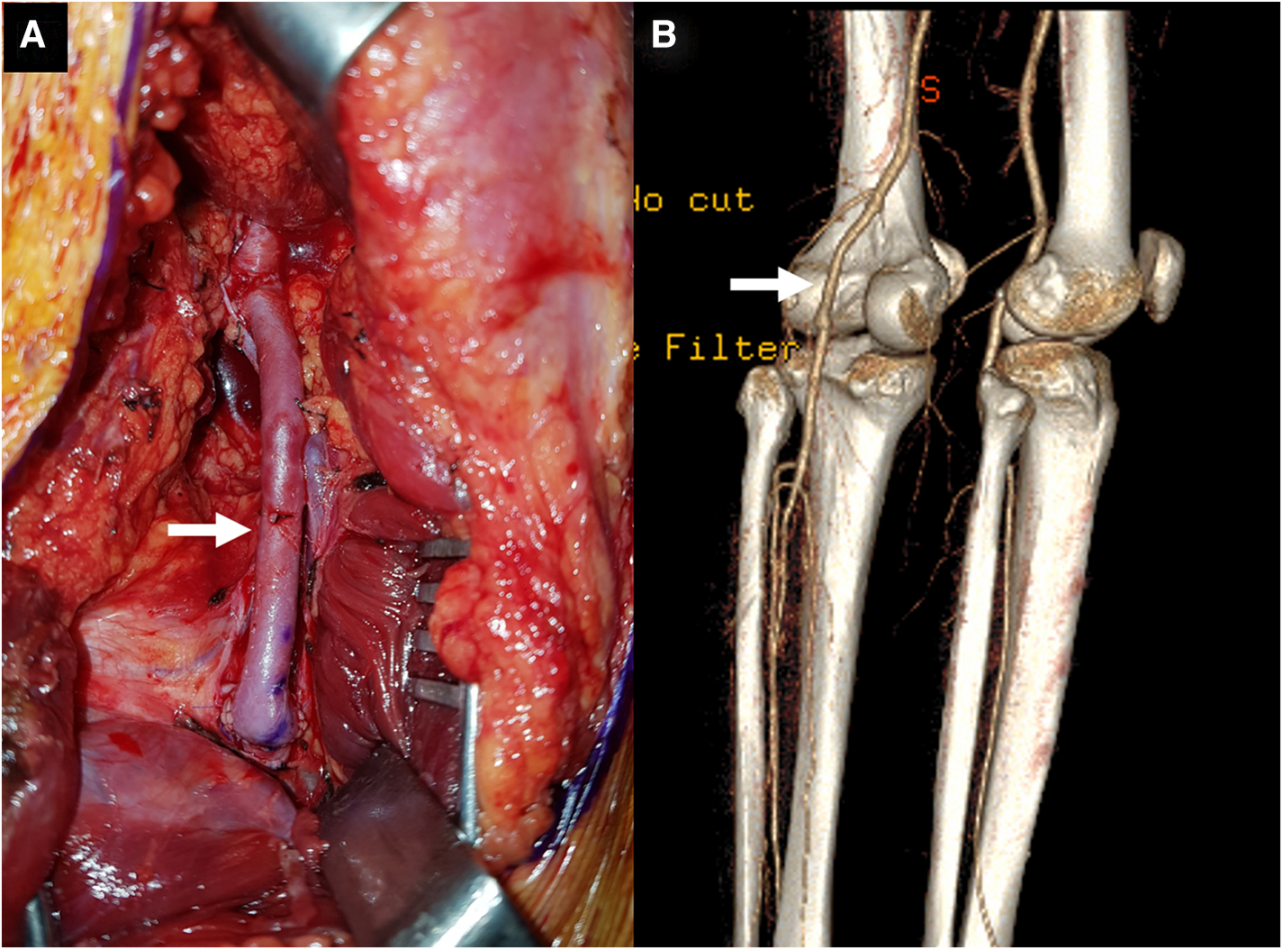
(A) The operative photograph showing a patent interposition graft (*arrow*) made using a reversed great saphenous vein at the distal thigh. (B) The follow-up computed tomography angiography showed a sufficiently patent graft (*arrow*) (posterior view).
